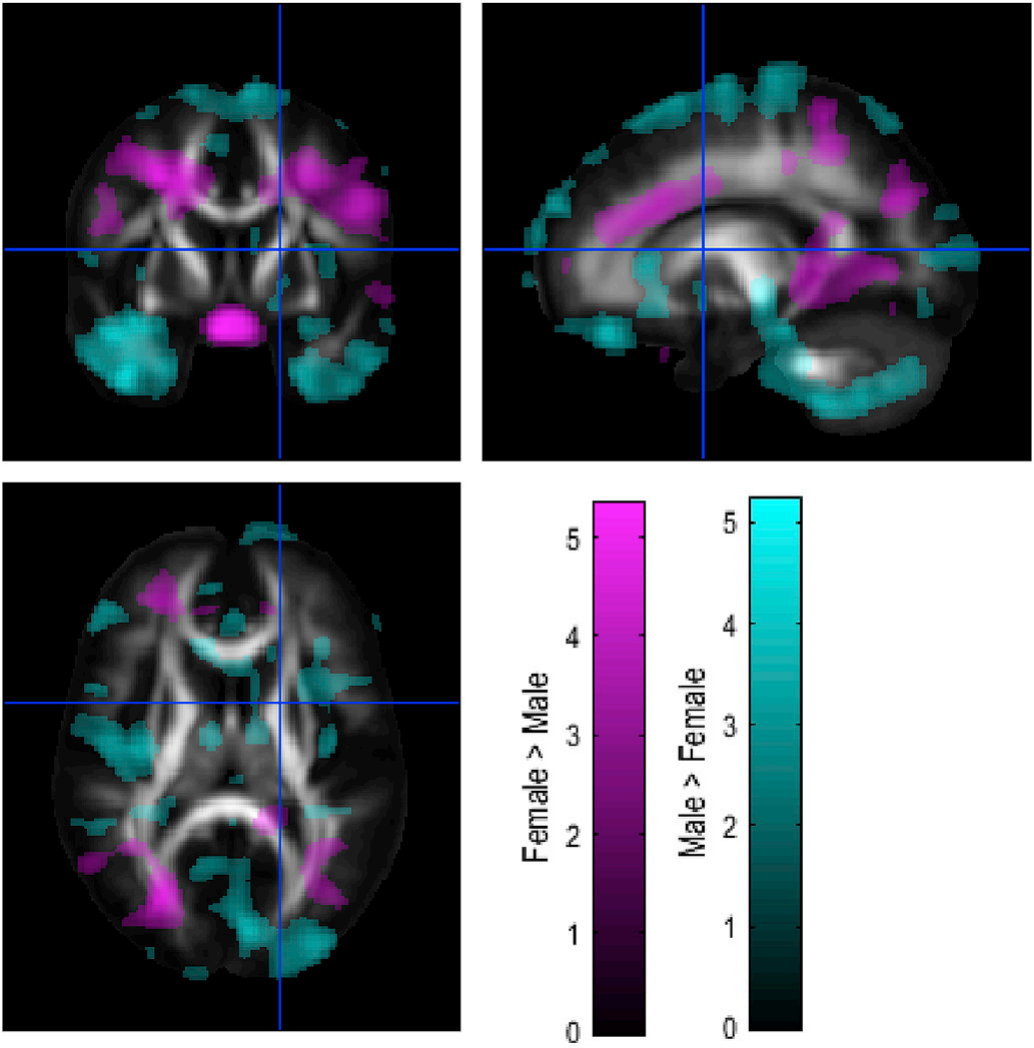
Regions showing sex differences in WM volume. Purple shows regions with greater WM volume in females. Turquoise shows regions with greater WM volume in males. T-statistic maps thresholded at p ​< ​0.05 uncorrected were overlaid on the average of individual T1-weighted images warped together into MNI space.
